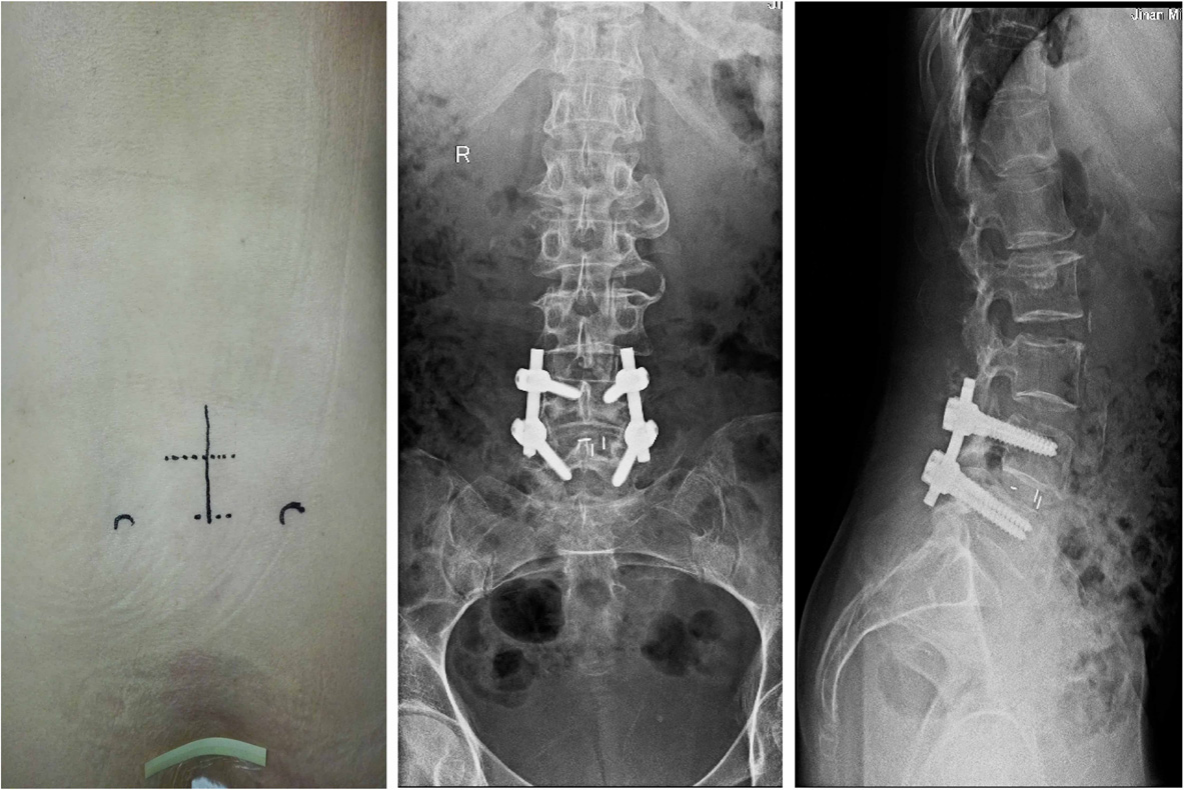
Anteroposterior and lateral CT scan showing MIS TLIF with BPS fixation in lumbar spinal fusion
